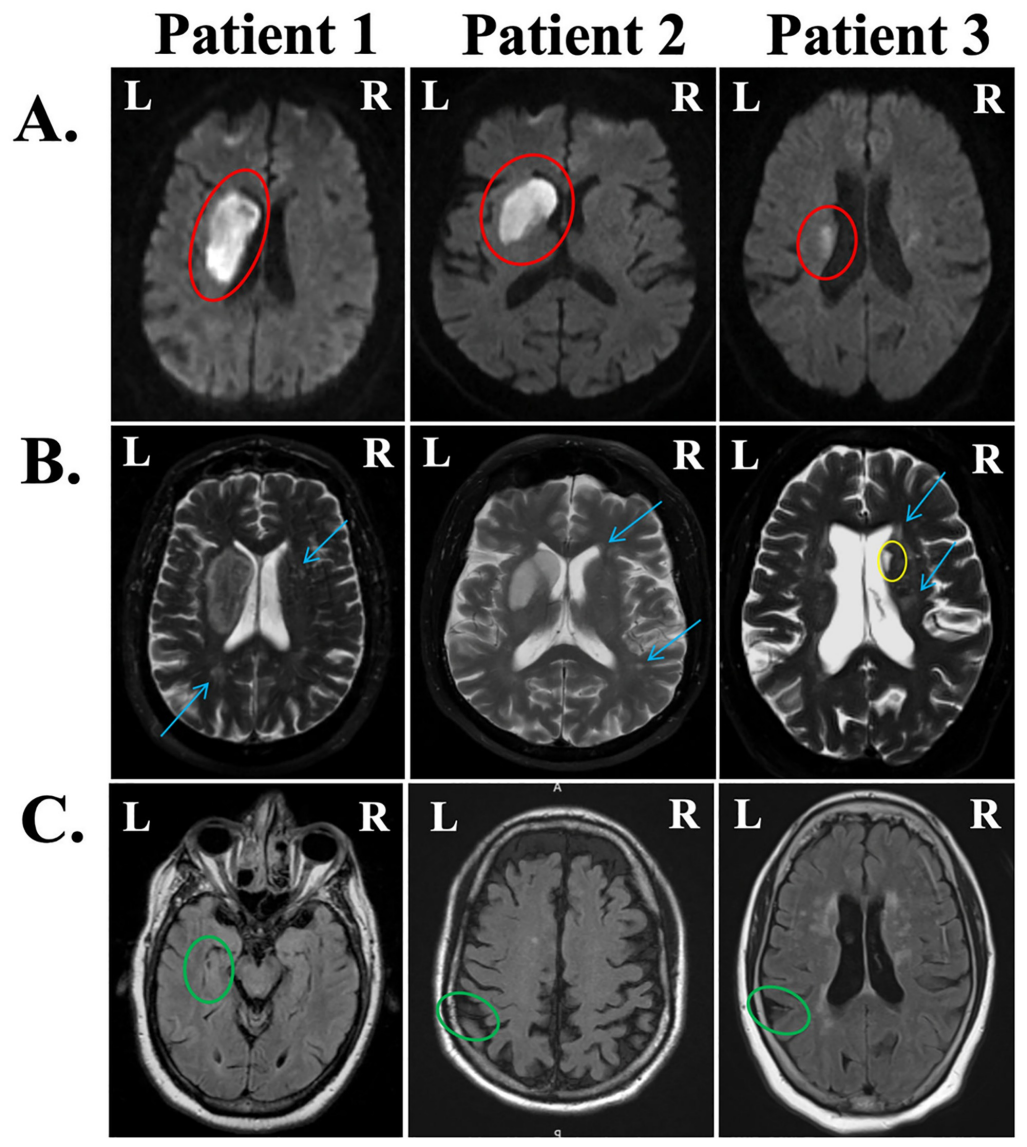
Clinical images from the three patients with marked language deficits in the acute phase. (A) Diffusion-weighted images (DWI) display acute lesions (red ovals). Patients 1 and 2 had lesions restricted to the basal ganglia, and Patient 3 had lesions to the basal ganglia and internal capsule. (B) T2-weighted scans were used to visually score leukoaraiosis on the Fazekas scale (blue arrows) from 0 to 3 and visualize chronic infarcts where present (yellow ovals). From left to right, periventricular hyperintensities (PVH) were scored as 2, 1, and 2; deep white matter hyperintensities (DWMH) were scored as 1, 1, and 2. (C) FLAIR hyperintense vessels (FHV) are indicative of hypoperfusion in areas surrounding infarcted tissue (green ovals). Only Patient 3 had a prior stroke. All three patients received FHV scores of 1 out of 12.
